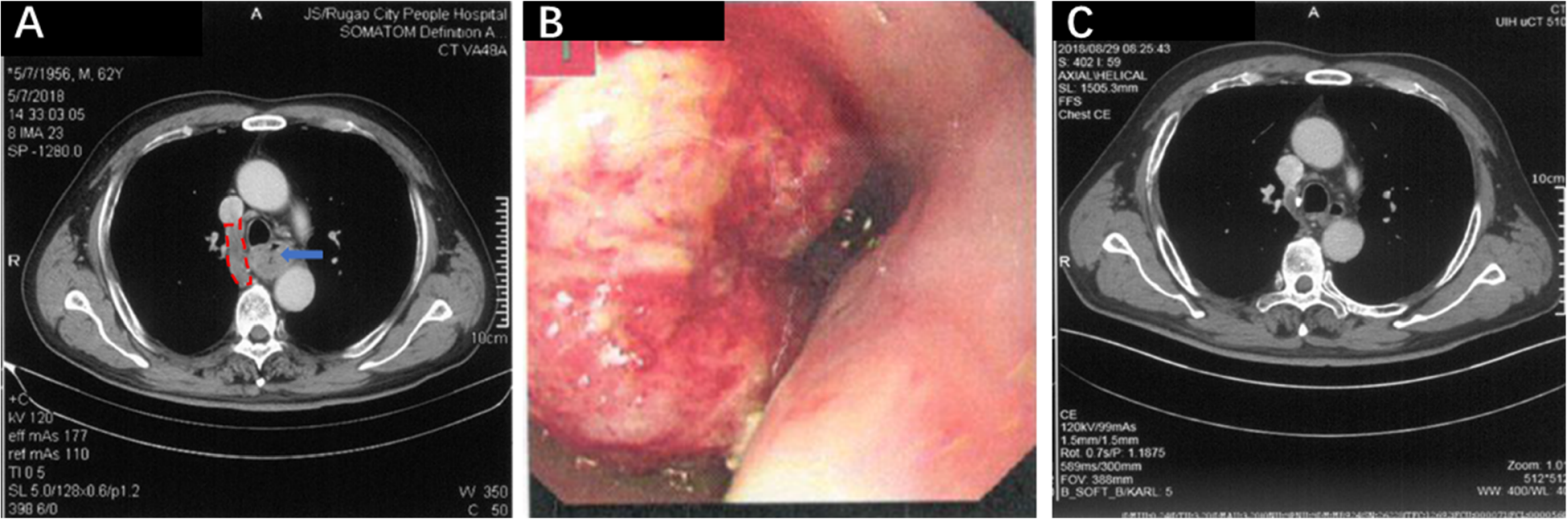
ESCC was diagnosed by CT and gastroscopy. a Chest enhanced CT before chemotherapy showed thicken walls of the middle esophagus with tumor thrombus in the arch of azygos vein. b Gastroscopic examination showed irregular protuberance in the esophageal lumen, and its surface remained anabrotic, and the tumor basically blocked the esophageal lumen. c Chest enhanced CT after neoadjuvant chemotherapy showed a significant reduction in the esophageal tumor and tumor thrombus at the arch of azygos vein
